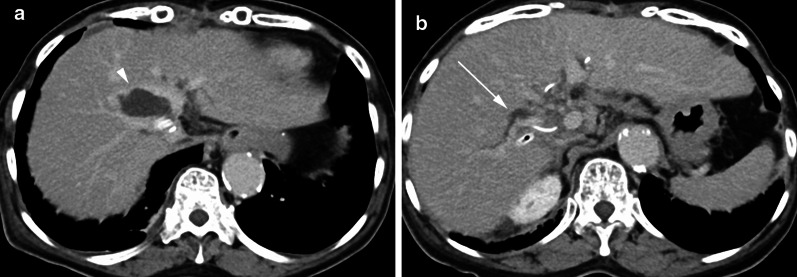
a Contrast-enhanced computed tomography after hepatectomy shows biliary leakage (arrowhead). b Intrahepatic bile duct of right lobe is dilated around the intraoperatively repaired site (arrow)
